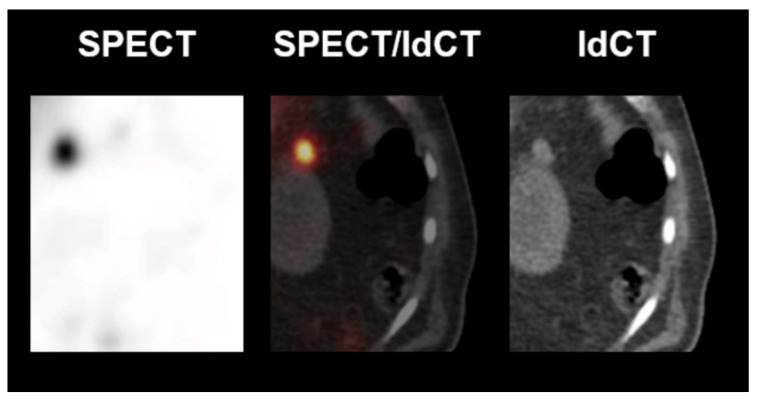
Example of a positive reading in spleen scintigraphy. Left anterior perigastric lesion. (Left) ^99m^Tc-labelled denatured red blood cells SPECT. (Middle) ^99m^Tc-labelled denatured red blood cells SPECT fused to low dose CT. (Right) low dose CT.
